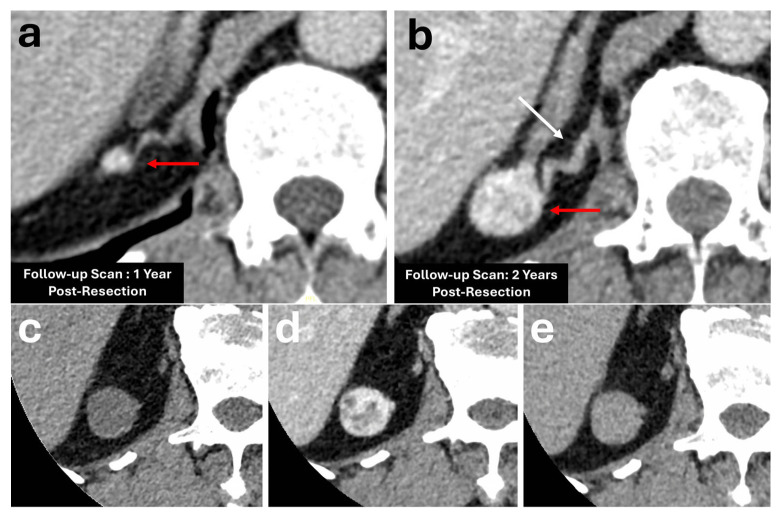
Patient with prior surgical resection of a functioning left adrenal adenoma. Follow-up CT examinations post-resection (a,b) show a gradually enlarging heterogeneous hypervascular nodule (red arrows) closely related to the right adrenal gland measuring 2.4 × 2.3 cm (previously 0.9 cm). A feeding vessel was noted on the anteromedial aspect of the lesion (white arrow). The nodule was 22 HU on the pre-contrast phase (c), 125 HU on the portal venous phase (d), and 70 HU on the delayed phase (e). Absolute washout was 53.4%. Relative washout was 44.0%. The patient remained asymptomatic with normal biochemical markers. Surgical resection was performed showing fibroadipose tissue with lobular aggregates of dilated blood vessels lined by bland endothelial cells. Mature adipose tissue and areas of hyalinisation were noted within the tumour. Findings were consistent with a haemangioma.
